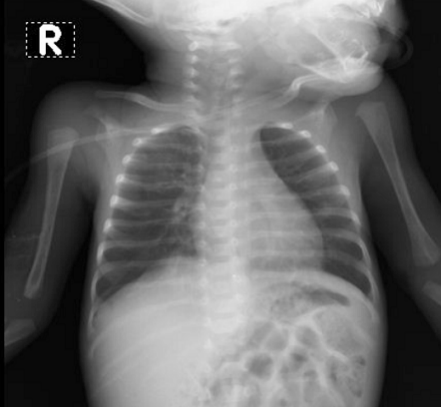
Patient 5 chest radiograph showing normal study
